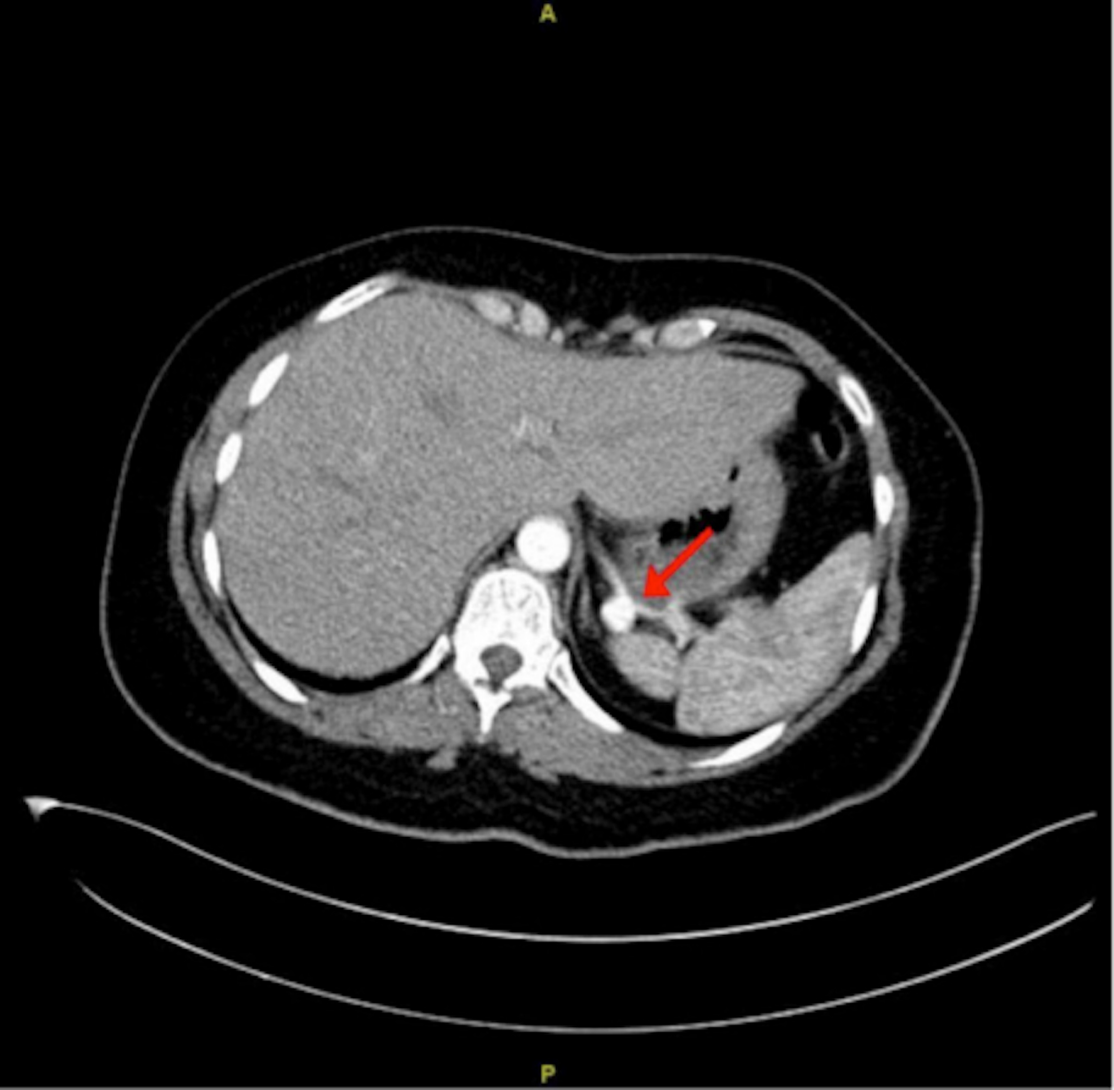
CT scan revealing an unruptured mid to distal splenic artery aneurysm
